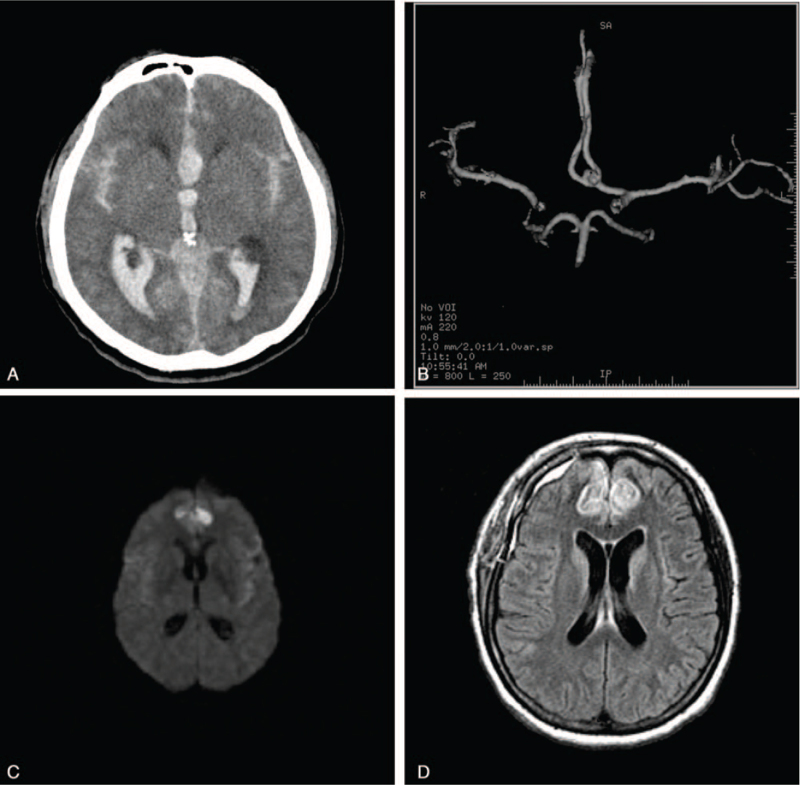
(A) Brain CT scans on admission showed a thick subarachnoid hemorrhage and global edema. (B) CTA revealed an anterior communicating artery aneurysm. (C) Postoperative diffusion-weighted imaging showed a high signal intensity area in the medial aspect of the bilateral frontal lobes. (D) Postoperative FLAIR image showing a high signal intensity area in the medial aspect of the frontal lobes. CT = computed tomography, CTA = computed tomographic angiography, FLAIR = fluid attenuated inversion recovery sequence.
